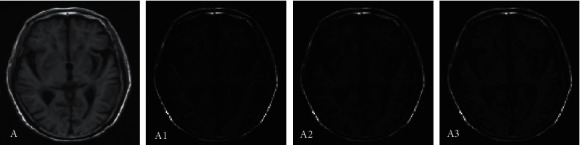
Comparison of MRI image segmentation at the top of the brain. Note: A represented the MRI image of the top of the brain; A1, A2, and A3 referred to the results of MRI image segmentation by CNN segmentation, FCN segmentation, and multimodal CNN, respectively.
